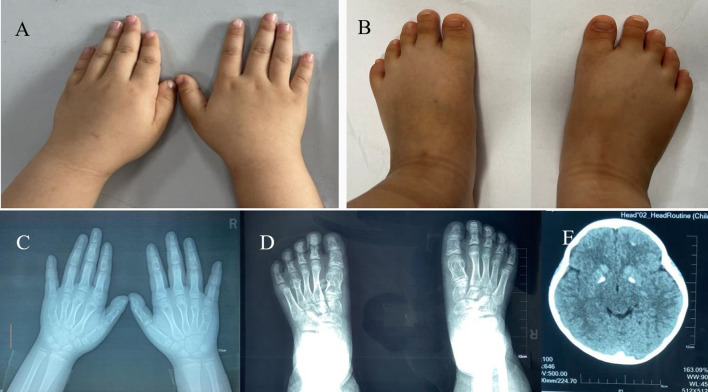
Appearance and imaging of proband 2. (A) Hand appearance, (B) foot appearance, (C) X-ray of both hands, (D) X-ray of both feet, (E) CT of the head.
